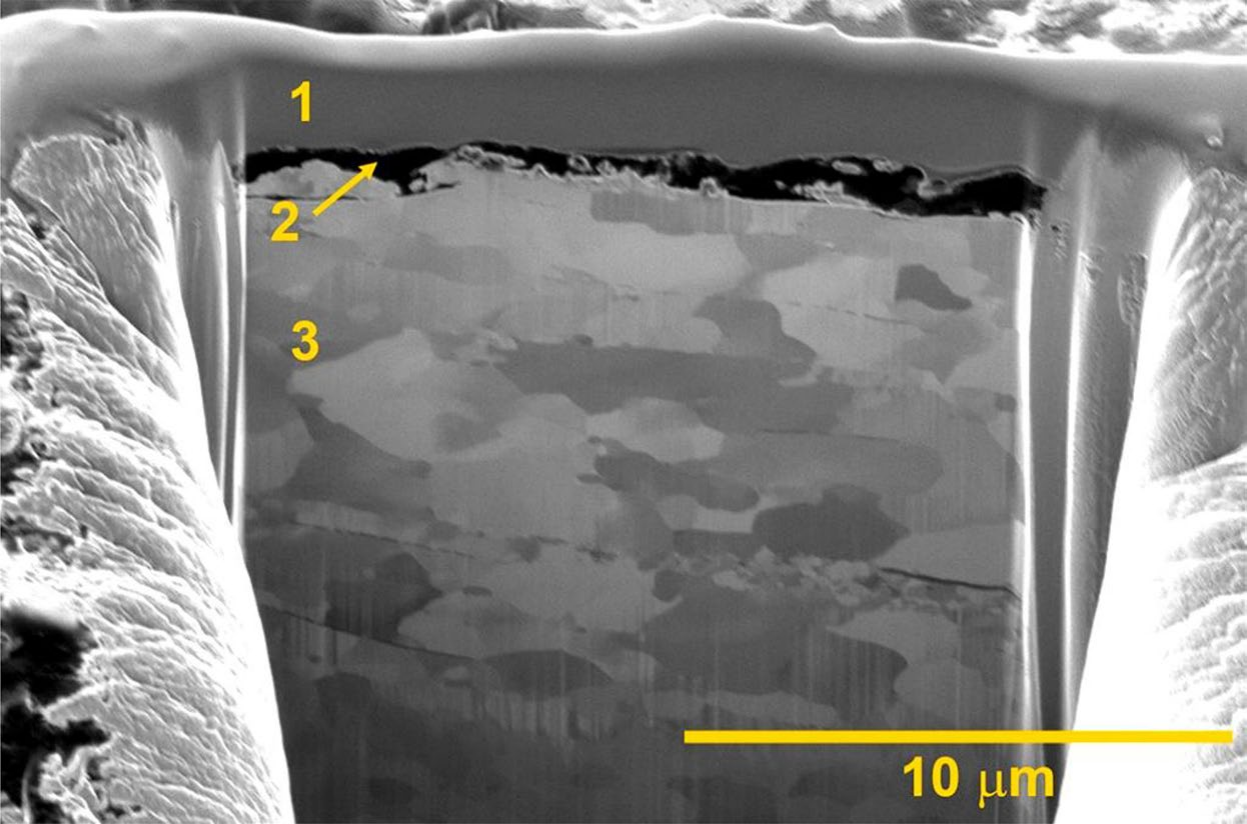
Focused ion bean (FIB) secondary electron image showing a cross-section of fragment #8 (Figure 6) recovered from the remains of flight crew member #2. Prior to imaging, the surface layers were exposed using an FIB. The elemental compositions of the layers were analysed using energy-dispersive X-ray microanalysis. 1) Protective layer of platinum deposited by the Netherlands Forensic Institute (NFI). 2) Layer of re-solidified cockpit glass. 3) Underlying steel.
